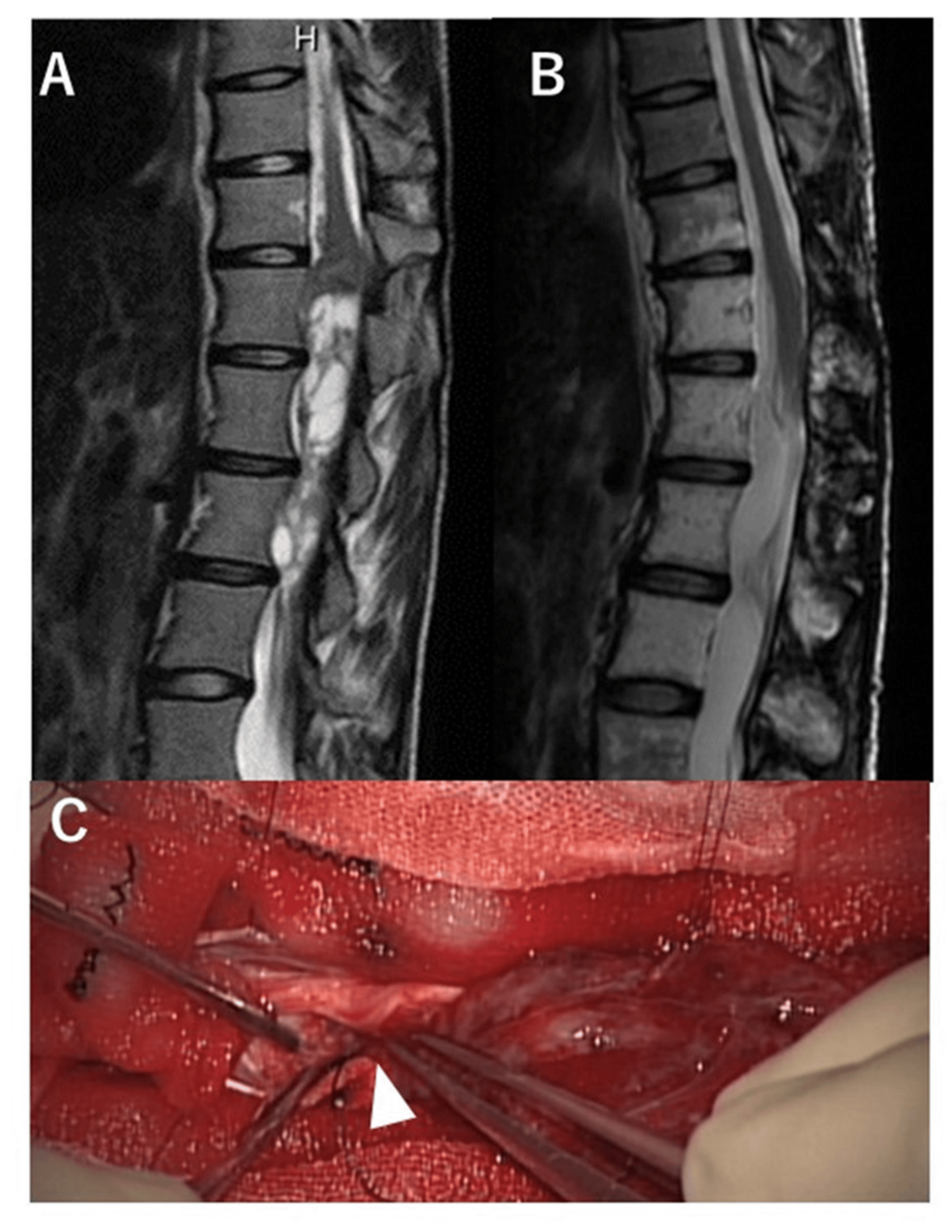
Pre- and postoperative MRI, intraoperative tumor finding. (A) A 21-year-old woman with an intradural tumor around the conus medullaris was shown in a preoperative sagittal T2-weighed MRI. (B) Seven years after postoperative sagittal T2-weighed MRI showed no tumor recurrence. (C) Intraoperative tumor findings showed that the tumor capsule had already ruptured and adhered to the conus medullaris.
